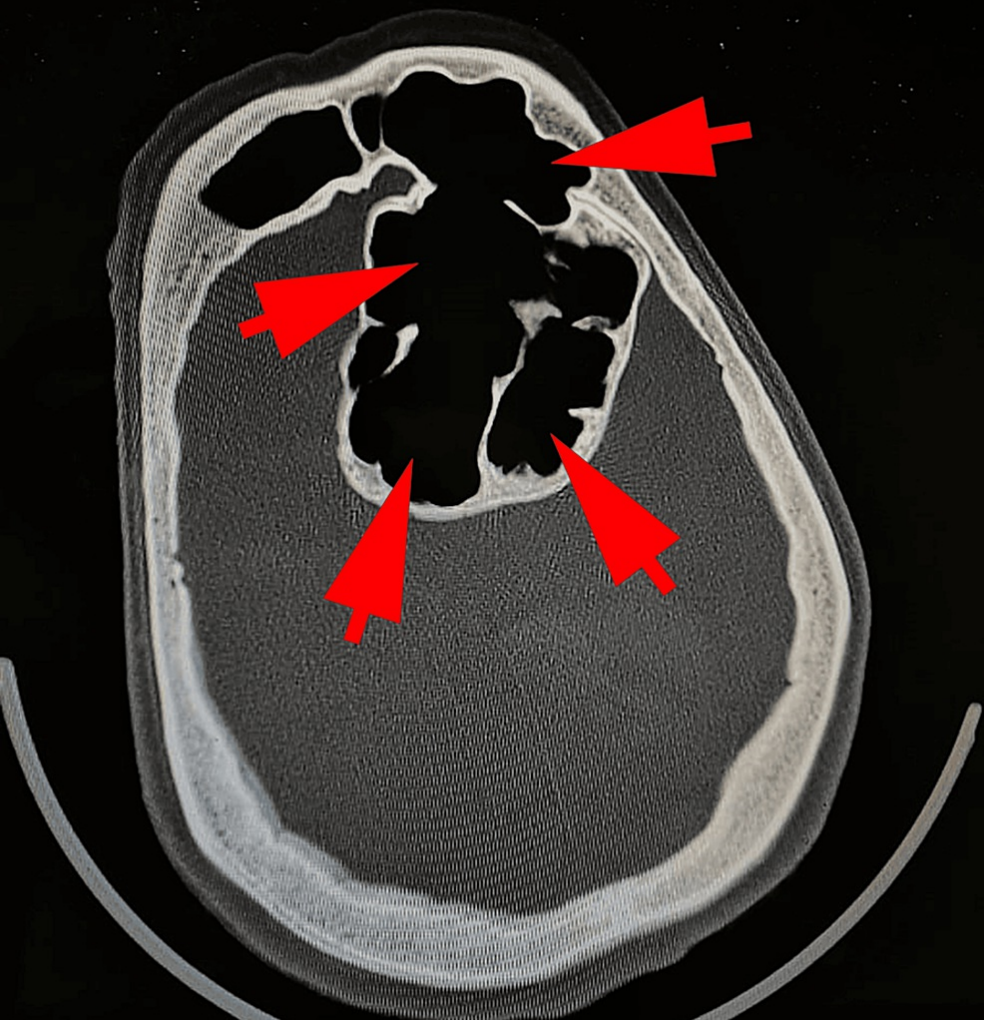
The computed tomography (CT) scan revealed significant enlargement of the frontal and ethmoidal paranasal sinuses (arrows), characterized by hyperpneumatization, consistent with PSD
